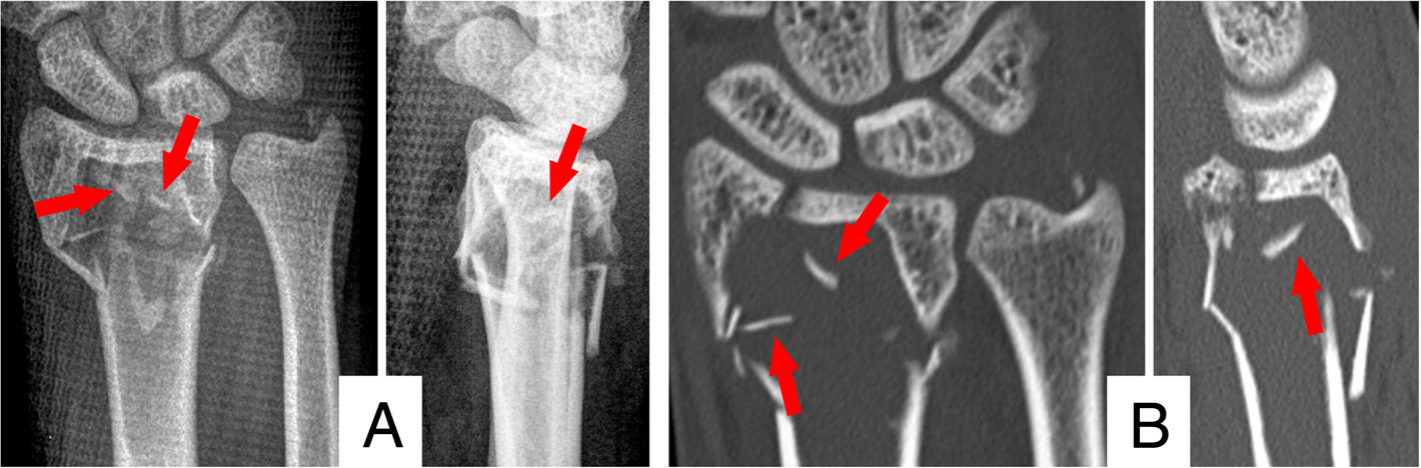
Summary of the radiographic findings. A) Plain radiographs of the wrist in two planes; B) Computed tomography of the distal radius; red arrows mark the ’fallen fragment sign’.
